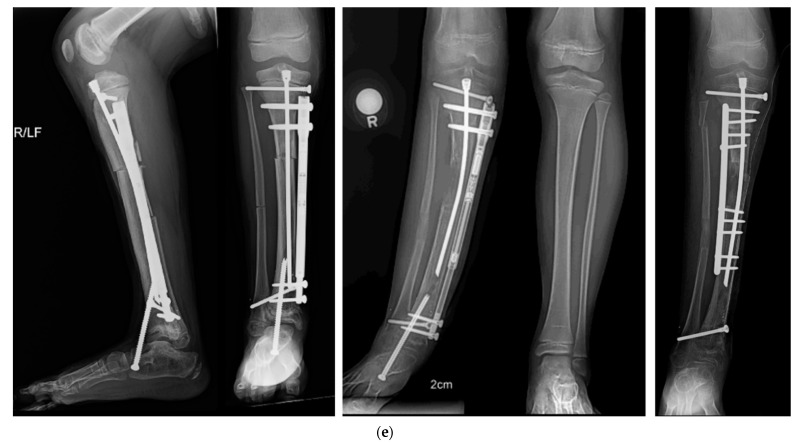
(a) AP and lateral radiographs of 18-month-old girl with Paley type 3b1 FH. She has a rocker bottom foot and obvious subtalar coalition malunion. (b) Intraoperative fluoroscopic views showing splitting of subtalar coalition with an osteotome (left) and after shortening of tibia relative to fibula with plate fixation (SHORDT) combined with subtalar coalition malunion reduction and pinning. (c) Radiograph showing lengthening of tibia with computer dependent external fixator at the same surgery as the SUPERankle procedure. A hemiepiphysiodesis plate was also placed to treat the distal femoral valgus. (d) Final radiographs after removal of external fixator and after correction of proximal tibial valgus with a hemiepiphysiodesis plate. Note the stable appearance of the ankle joint and the plantigrade foot position. (e) Radiographic sequence of extramedullary lengthening with medially placed Precice nail (Nuvasive Specialized Orthopedics, California) (left). There is a Simple Locking IntraMedullary (SLIM) rod (Pega Medical, Montreal, Canada) and the fibula is fixed with tibio-fibular screws. The foot is fixed with a temporary extra-articular spanning screw from the foot to the tibia anterior to the ankle joint. A 5 cm lengthening was performed causing axial deviation into valgus bending the SLIM rod (middle). To correct the valgus, a plate was inserted laterally, and the extramedullary nail removed, after first decompressing the peroneal nerve, performing an anterior compartment fasciotomy and cutting the fibula proximally (right).
